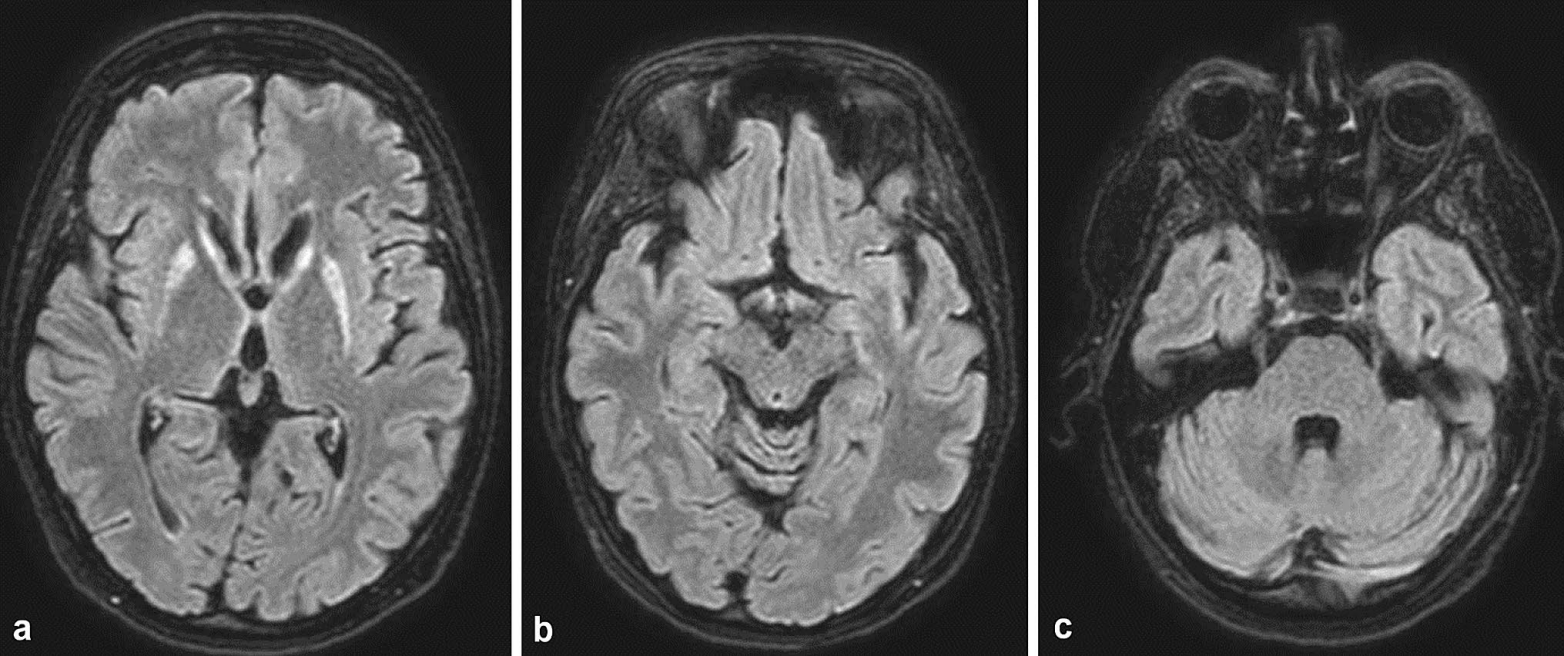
Axial T2-FLAIR shows bilateral hyperintensities in the putamen and caput nuclei caudati (a), but sparing (b the dorsomedial thalami, periventricular areas around the 3rd ventricle and mesencephalic aqueduct, mammillary bodies)
